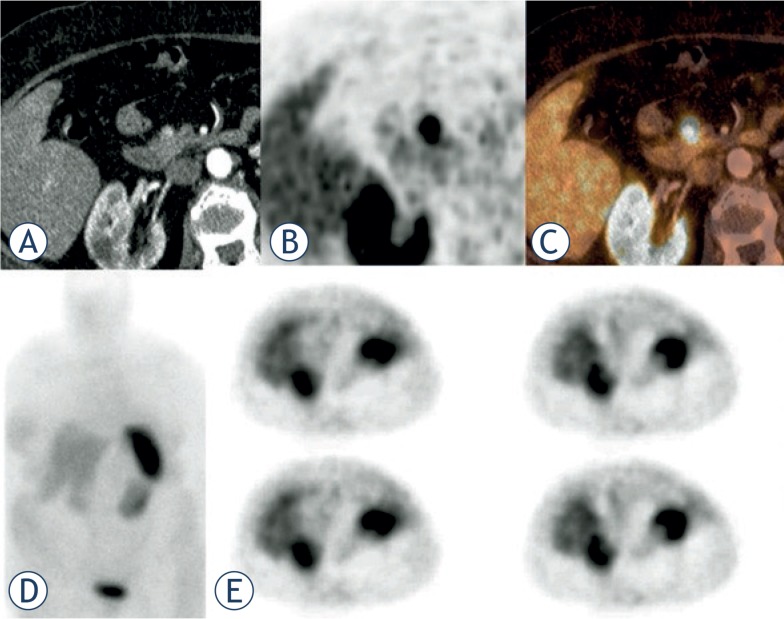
(A–C) Insulinoma presenting as a hyperperfused (A) Ga-68 DOTATOC positive lesion (B) in the pancreatic head (C). In the In-DTPA octreotide scintigraphy (D) and SPECT (E) performed 3 days earlier no lesion could be found.
